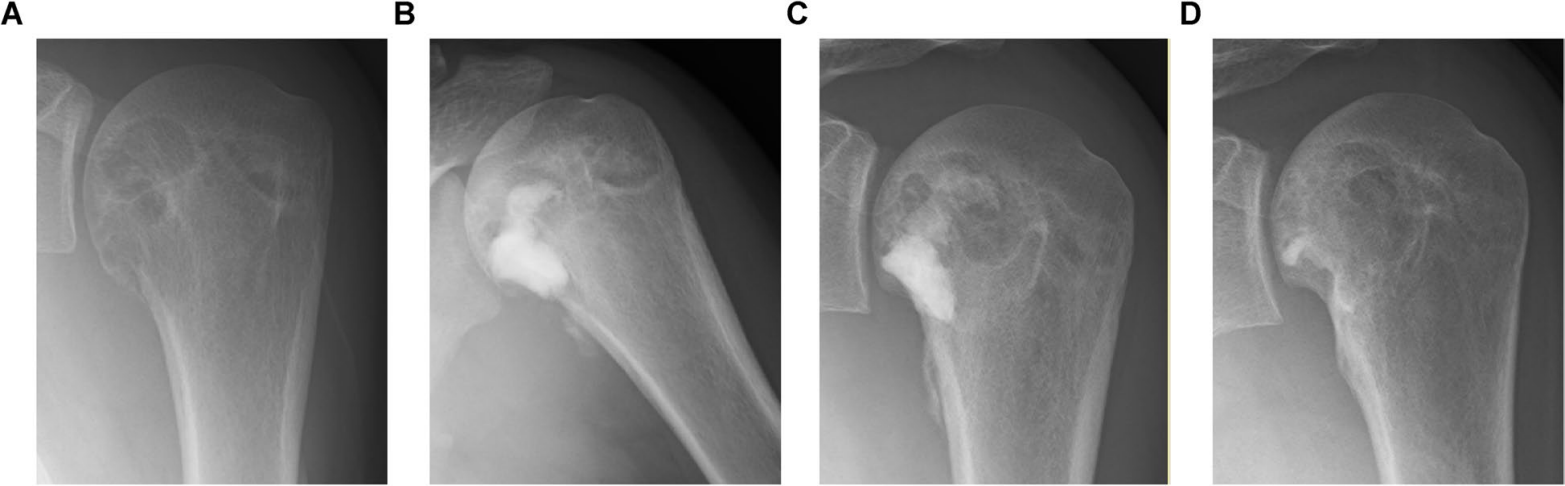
Radiographs of a chondroblastoma in the proximal humerus. Radiographs at presentation (A), initial surgery (B), time of local recurrence (C), and last follow-up (D). A 16-year-old boy presented with chondroblastoma of the proximal humerus. Curettage and ablation were performed without using high-speed burrs during the first surgical procedure. The patient underwent artificial bone grafting. Seven months after the surgery, the patient's symptoms flared, and the translucent image near the joint enlarged. The patient underwent another curettage procedure, and artificial bone grafting was performed. Seven months after the reoperation, there was no apparent local recurrence. The Musculoskeletal Tumor Society (MSTS) score was 30, although a slight degeneration was observed from the proximal humerus to the neck
